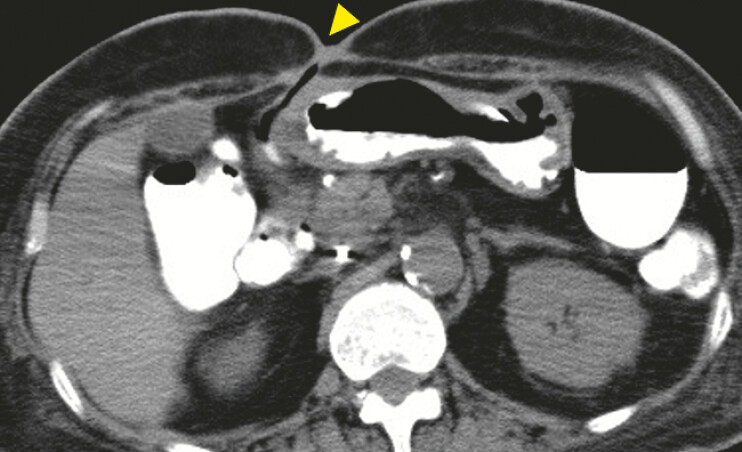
Abdominal CT image after reoperation. Abdominal CT showed the duodenocutaneous fistula persisted after reoperation. (△) showed the persisted duodenocutaneous fistula. Abbreviation: CT, computed tomography.
